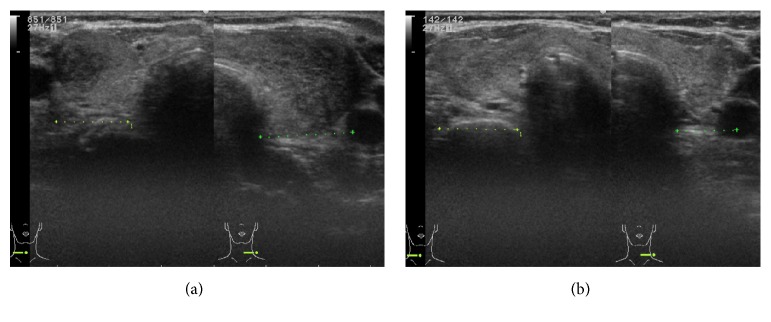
Ultrasonography of the thyroid. (a) Heterogeneous and hypoechoic areas were observed in both thyroid lobes before treatment. (b) Hypervascularity and a reduction in hypoechoic areas, indicating improvement, were observed 10 weeks after treatment initiation.
